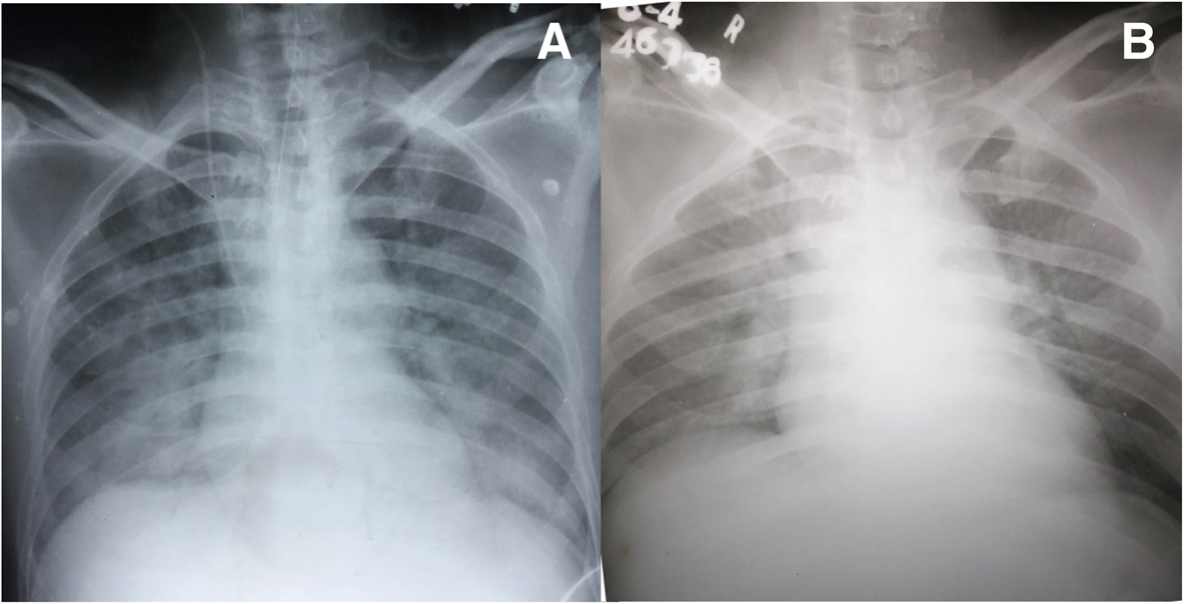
Chest Radiographs. A - Chest radiograph on day 2 of the illness with bilateral diffuse alveolar shadows. B - Chest radiograph at the time of discharge with clearing of alveolar shadows
